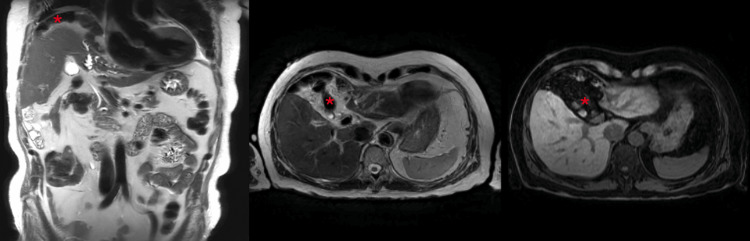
MRI performed as a follow-up of the CT scan confirming the Chilaiditi sign. The asterisk (*) marks the bowel segment situated above the liver.
